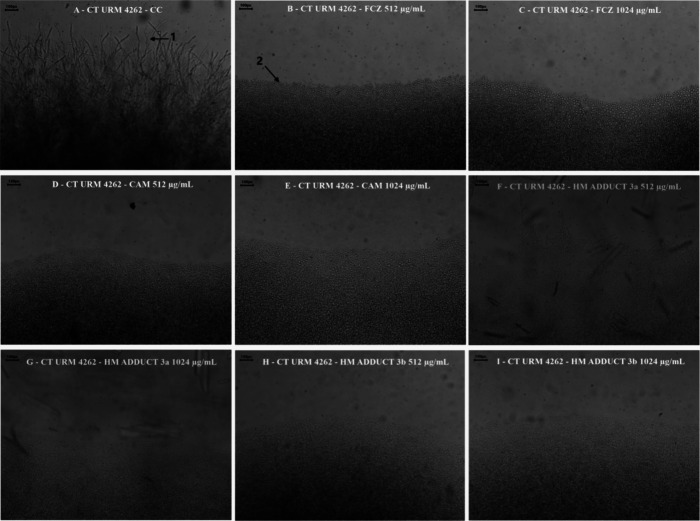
Morphological transition of *Candida tropicalis*. CT: *Candida tropicalis*; URM: University
